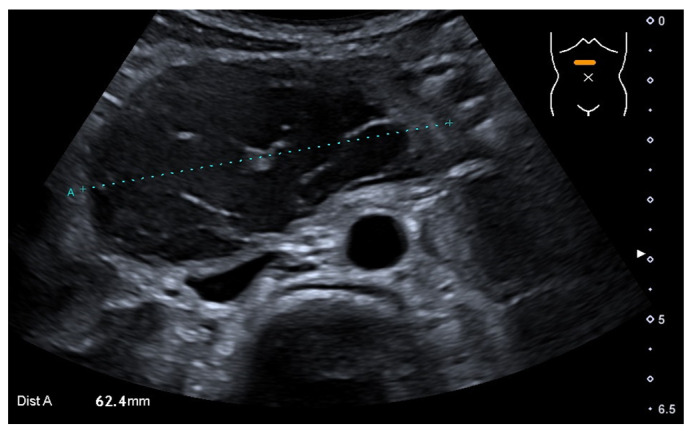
Initial abdominal ultrasound with intramural hematoma of the duodenum (dotted line), which completely compressed its lumen.
